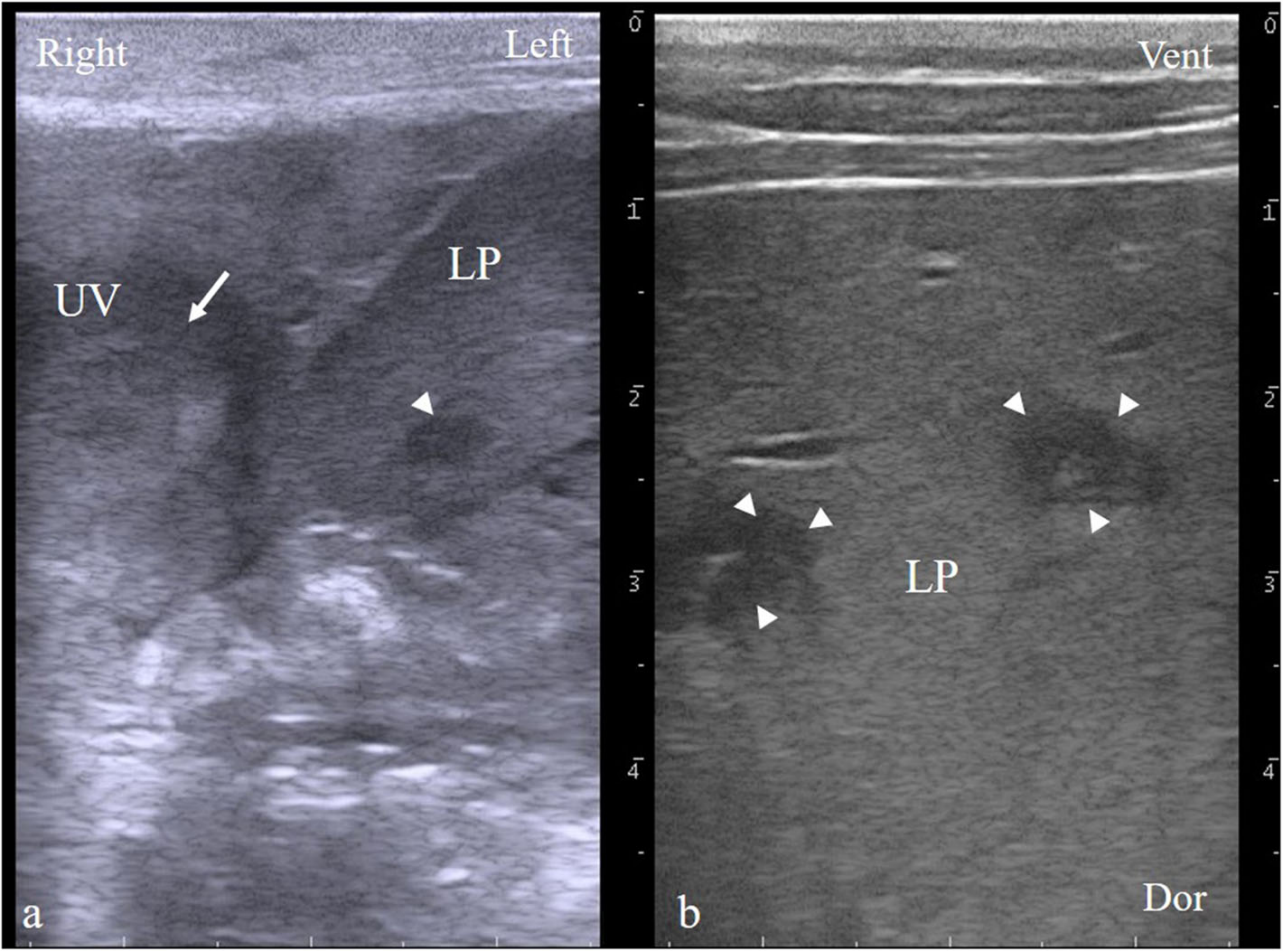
Ultrasound imaging (transverse plane) with a 10.0-MHz liner probe demonstrating the infected umbilical vein. a Scanner placement cranial from the abdominal fundus. The umbilical vein showed a horizontal diameter of 25 mm and a vertical diameter of 30 mm. The lumen of the vein had an area of relatively high echogenicity (arrow). b Scanner placement at the right 11^th^ intercostal space showing a hepatic abscess larger than 1 cm (arrowhead). LP, liver parenchyma; UV, umbilical vein; Vent, ventral; Dor, dorsal
